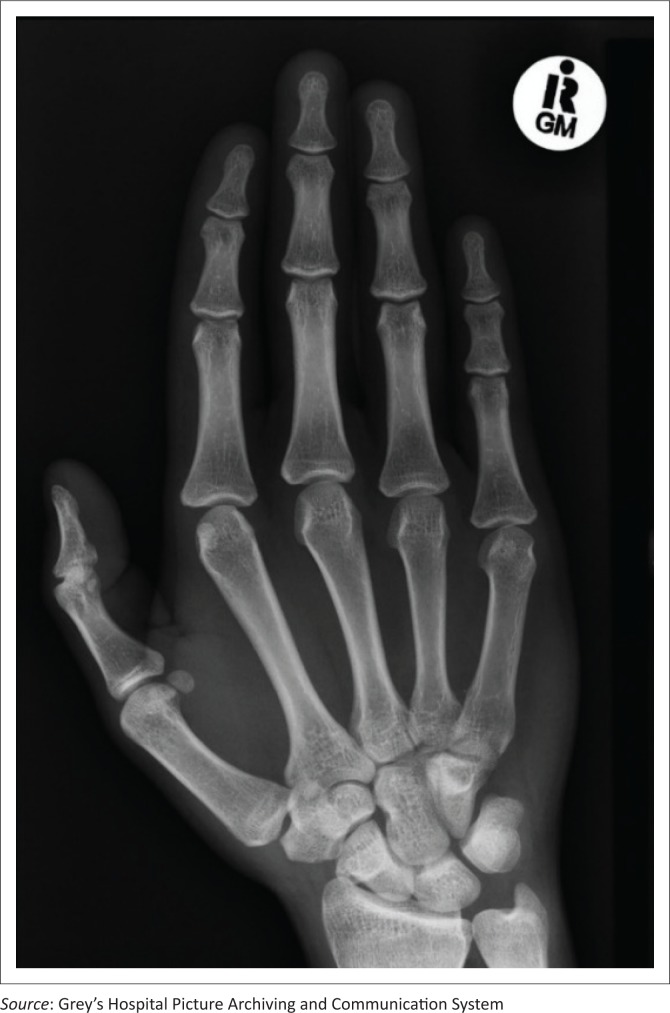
Digital hand-wrist radiograph (right) of a female subject with a chronological age of 20 years 8 months was matched to the GP standard image of a 17-year-old by both readers.
